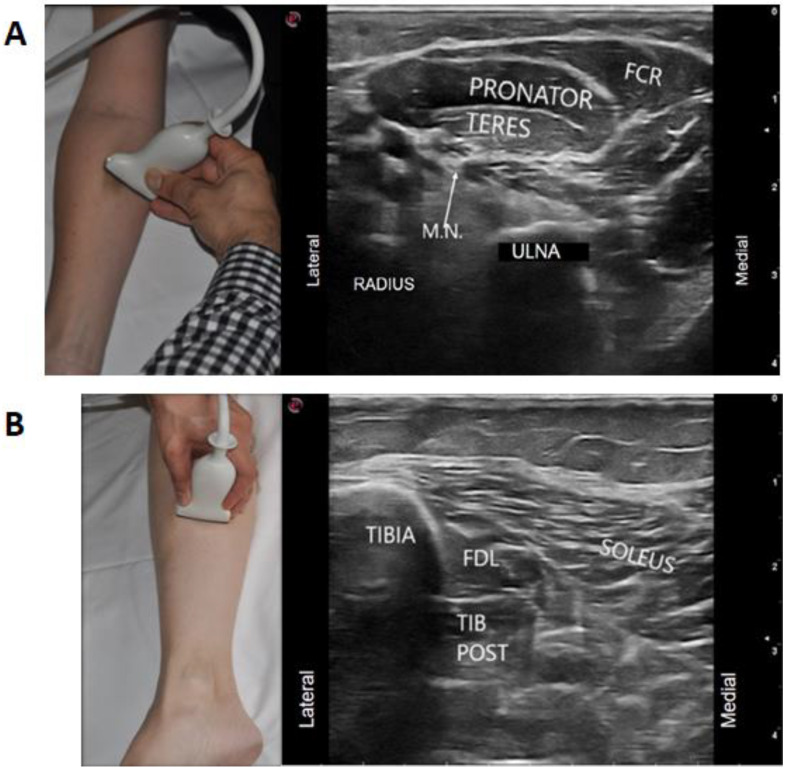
Visual ergonomics. (A) Positioning transducer one transversal length down from the cubital fossa offers a scouting landmark: FCR can be recognized as a “shark eating a ball”, in this case, the ball referring to pronator teres. (FCR: Flexor Carpi Radialis, PT: Pronator Teres, M.N.: Median Nerve). (B) Flexor digitorum longus having an appearance of a “sail” or “shark-fin” just lateral to the tibia can be used to identify other muscles such as the tibialis posterior separated by a fascial plane just underneath. (Images acquired on Esaote MyLab 7 ultrasound machine, Esaote ultrasound machine with 13–3 Hz linear array transducer, (Esaote; location: Fishers, IN, USA)).
